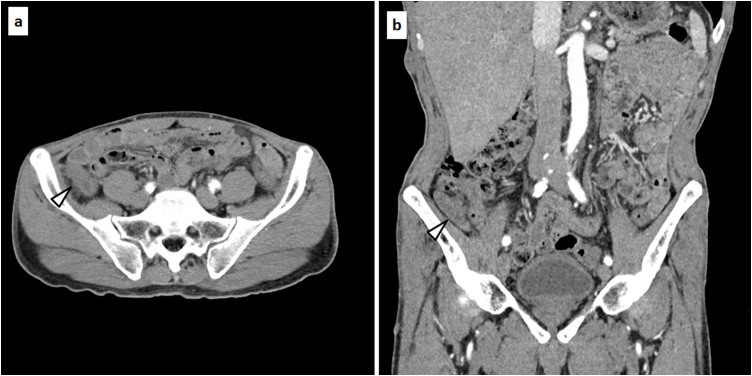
Contrast-enhanced CT showed an enlarged and diffusely thickened appendix (appendix: thick white arrow head).
